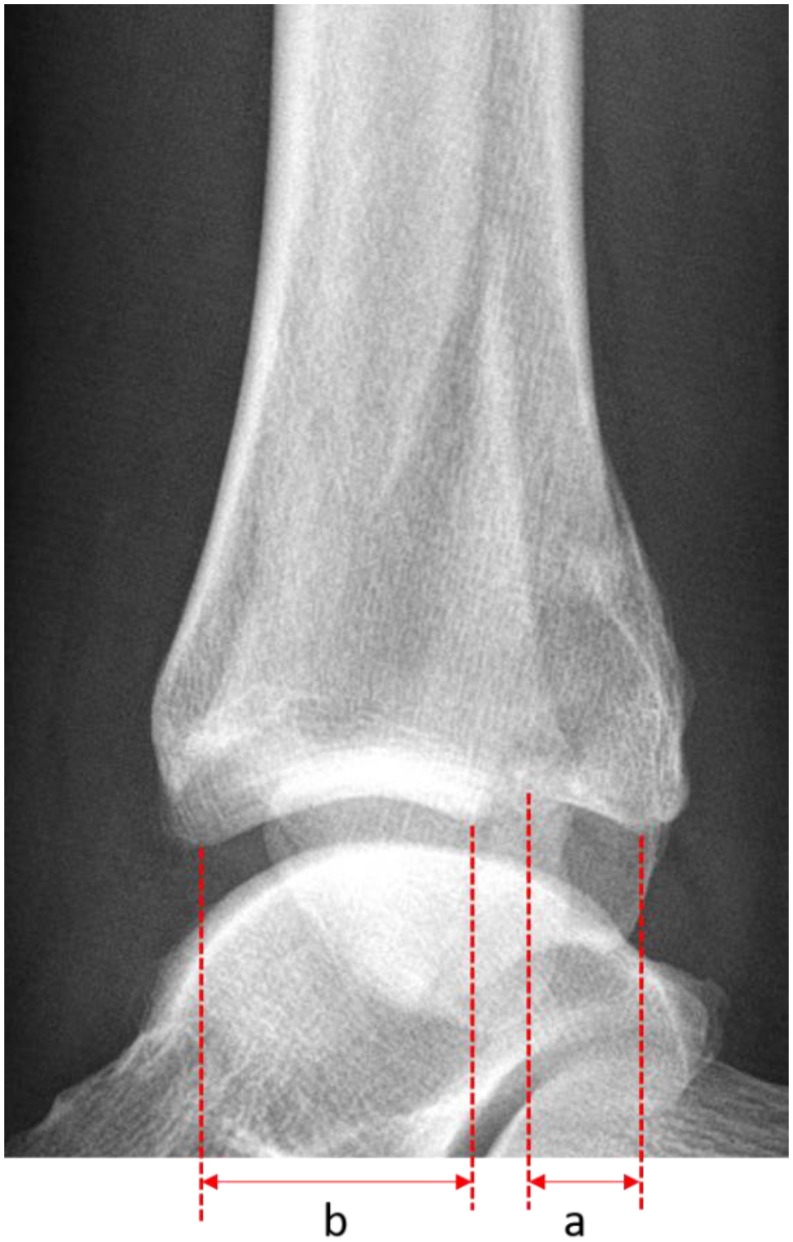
The size of the PMF is measured in the lateral X-ray and divided by the total articular surface then expressed in percent
